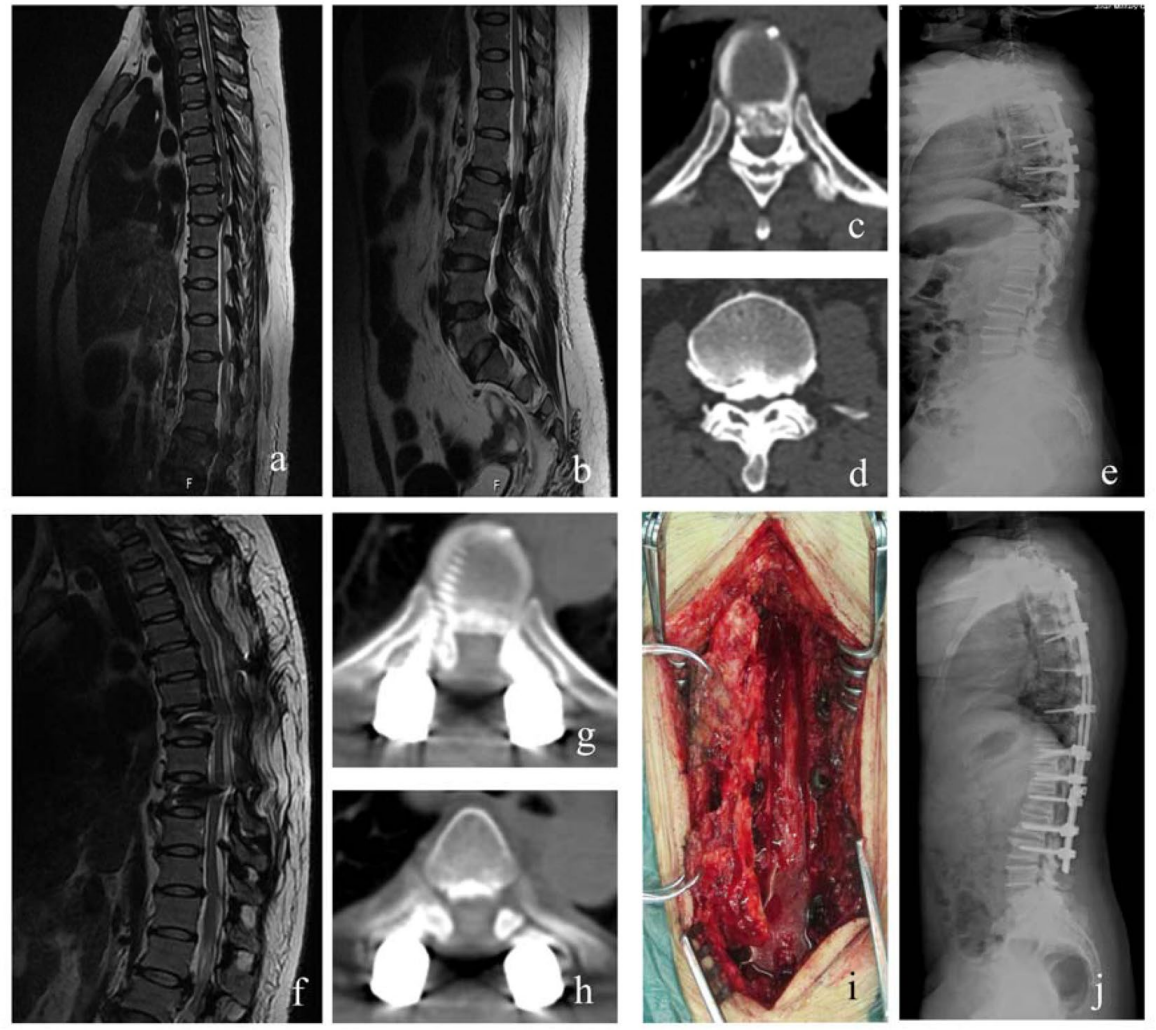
Coexisting ossified posterior longitudinal ligament (OPLL) and ossification of the ligamentum flavum (OLF) involving thoracic and lumbar levels treated with staged laminectomy using the ultrasonic device A 49-year old female patient exhibited paraplegia (JOA, 4), magnetic resonance images (MRI) showed concomitant OPLL and OLF involving T2–9 and T10-L2 levels (a,b); computed tomography (CT) indicated severe spinal canal occupancy at thoracic (c) and lumbar (d) levels; the patient underwent thoracic laminectomy first (e), 12 months after operation, ideal thoracic decompression was demonstrated by MRI (f) and CT (g,h); she had second operation for OPLL and OLF in T10-L2 levels at an interval of 12 months (i,j). She had a 46.2% improvement rate 30 months postoperatively.
